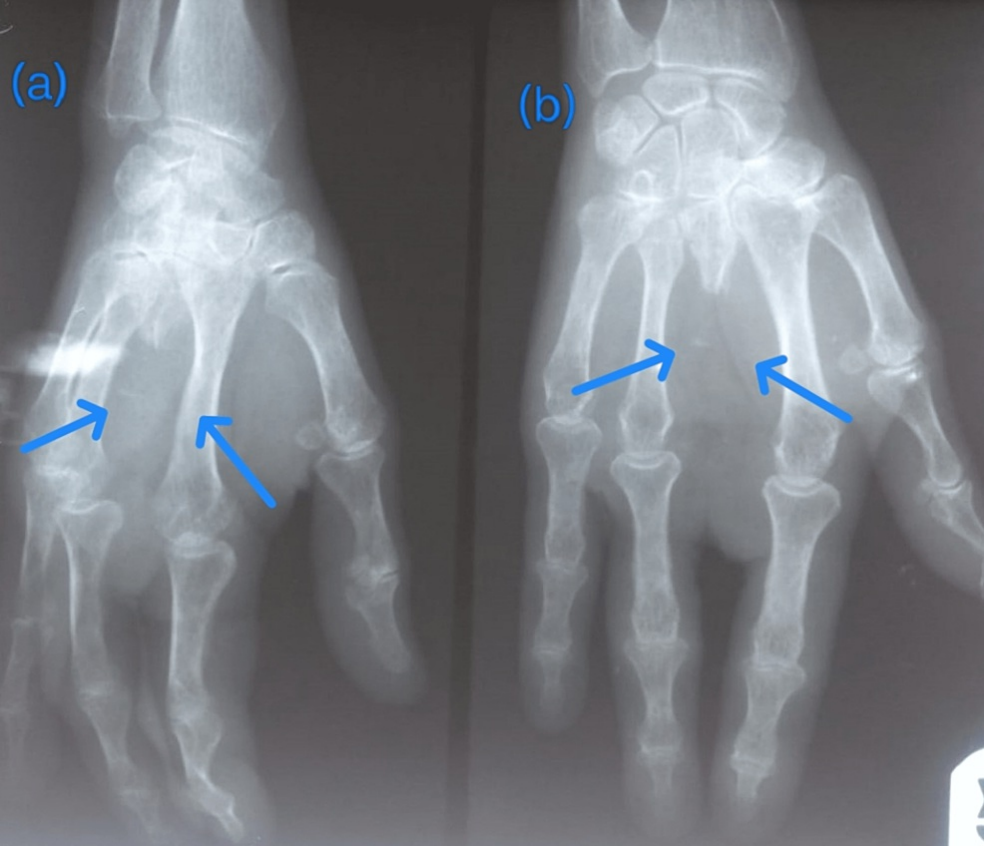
Case 2: postoperative X-ray
